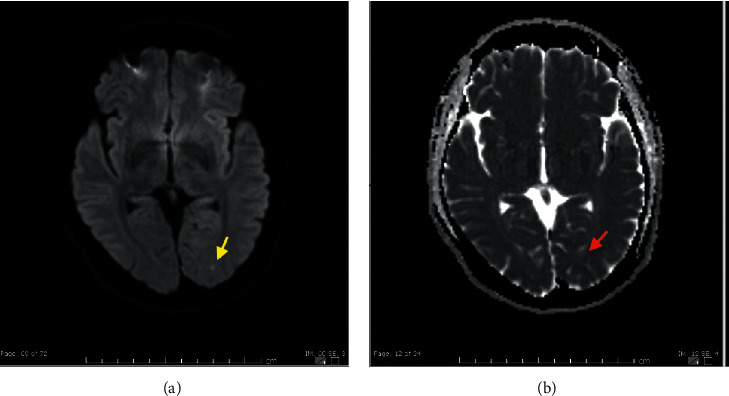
(a) Diffusion-weighted imaging (DWI) MRI head showing a tiny occipital subcortical hyperintense lesion. (b) The same lesion appearing faintly hypodense on apparent diffusion coefficient (ADC) MRI head. These findings of diffusion restriction were keeping with acute to early subacute lacunar infarction. Notably, these findings were not present on the new MRI done in the third admission (index admission).
